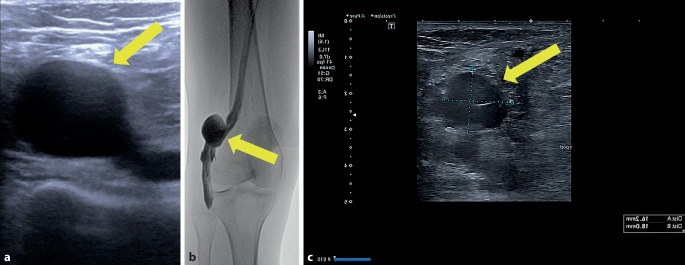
Fall 1: Venöses Aneurysma der V. poplitea rechts. a Sonographische Darstellung des Aneurysmas der V. poplitea im B‑Bild (*gelber Pfeil*), b Phlebographische Darstellung des venösen Poplitealaneurysmas rechts (*gelber Pfeil*), c Postoperative Ultraschallkontrolle des versorgten Aneurysmas der V. poplitea im Querschnitt mit 1,6 cm (*gelber Pfeil*)
